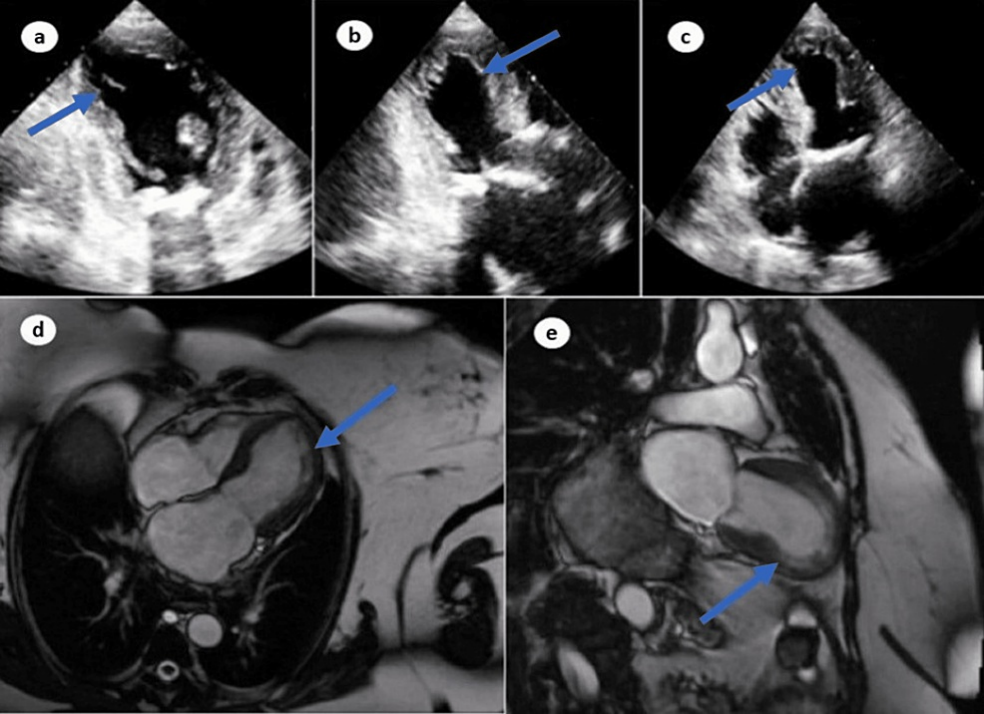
Echocardiogram revealing apical ballooning of heart (a-c) and cardiac ventriculogram revealing apical ballooning ruling out myocarditis (d, e).
